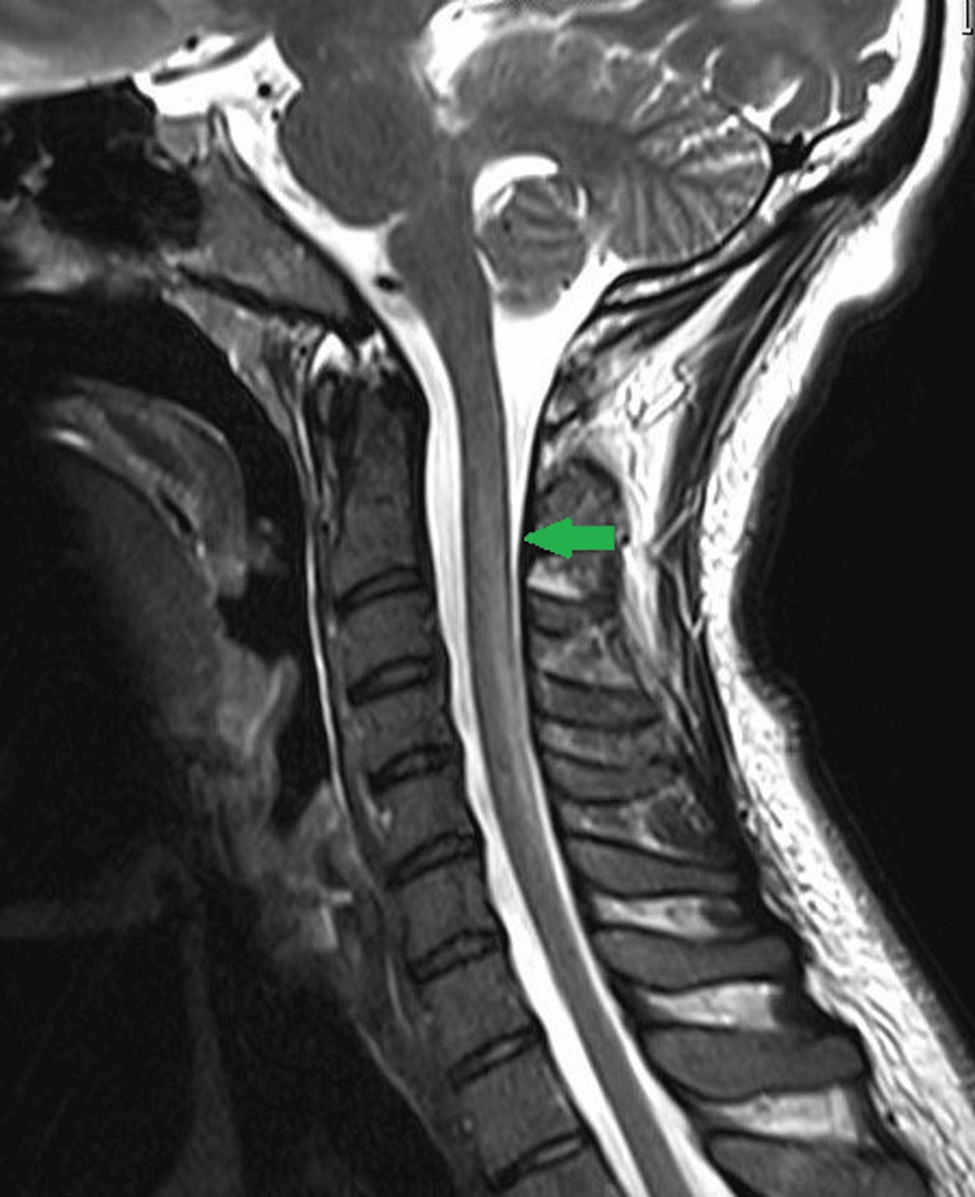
MRI cervical spine T2 weighted image (sagittal view) T2 hyperintense signals can be seen within the posterior part of the cervical cord, extending from C2 to C6 vertebral levels and associated with mild swelling of the cervical cord (green arrow). MRI, magnetic resonance imaging
